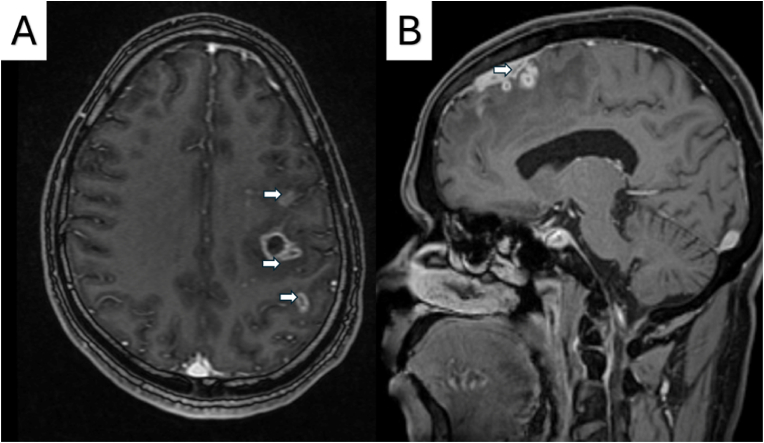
Axial and Sagittal sections of magnetic resonance imaging in patients with neuromelioidosis showing the tunnel sign (white arrow).
